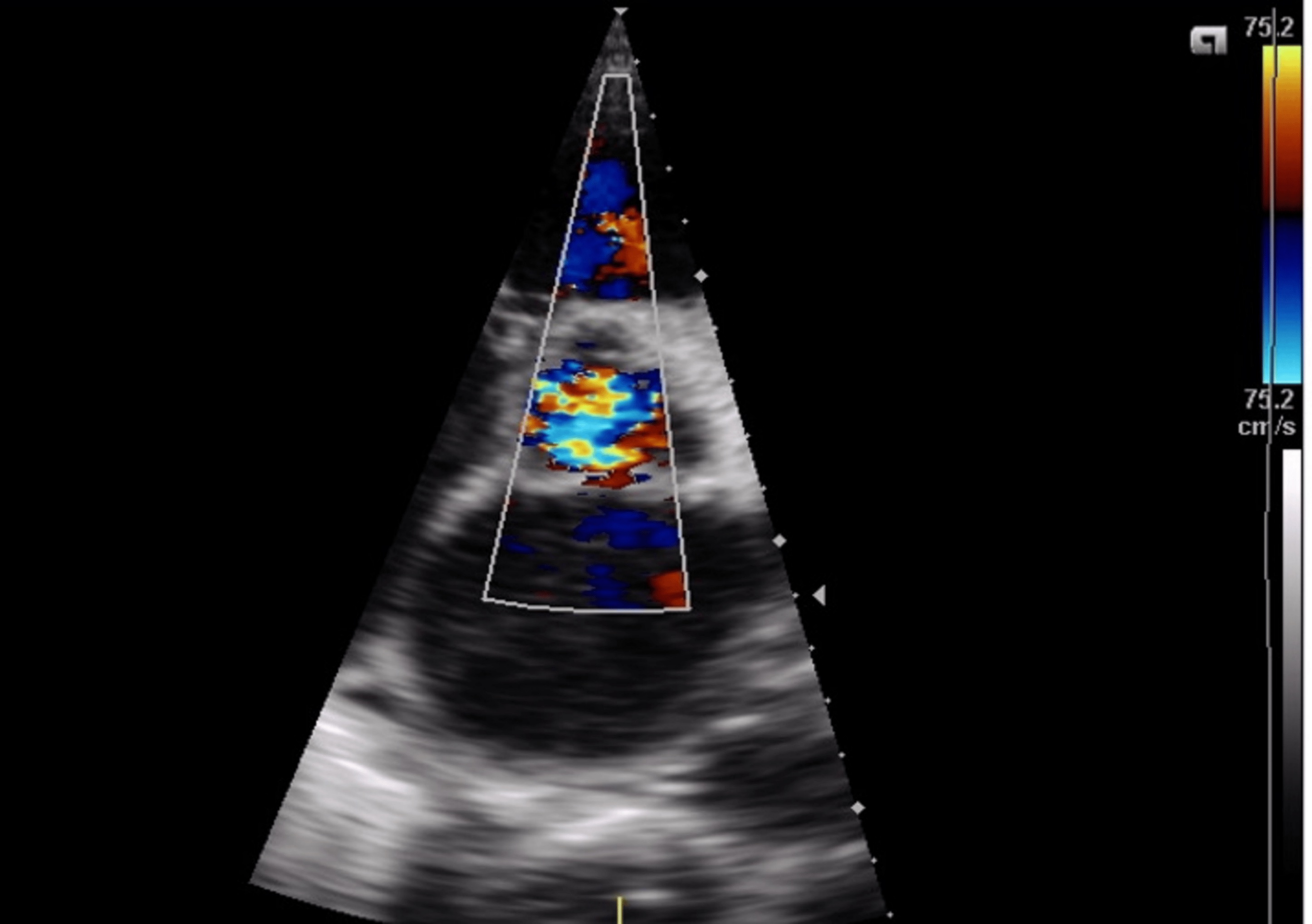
Parasternal short-axis view of the aortic valve with color Doppler Imaging showing severe aortic insufficiency
